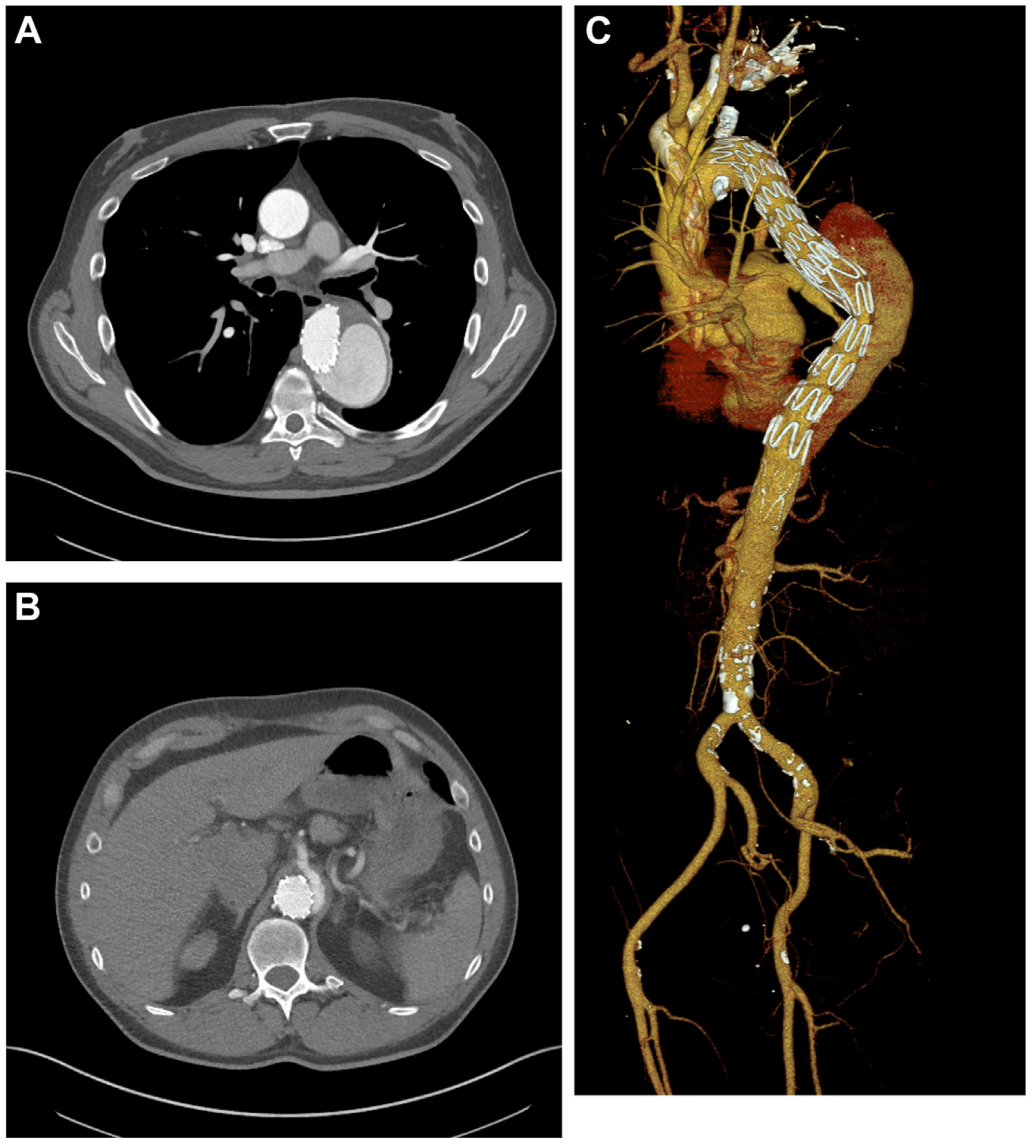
Preoperative images. (A) Axial image of aneurysm at the descending aorta with prior thoracic endovascular aortic repair (TEVAR) graft. (B) Bare-metal stent at the level of the celiac artery with chronic dissection. (C) Three-dimensional reconstructed image of the computed tomography angiogram (CTA).
